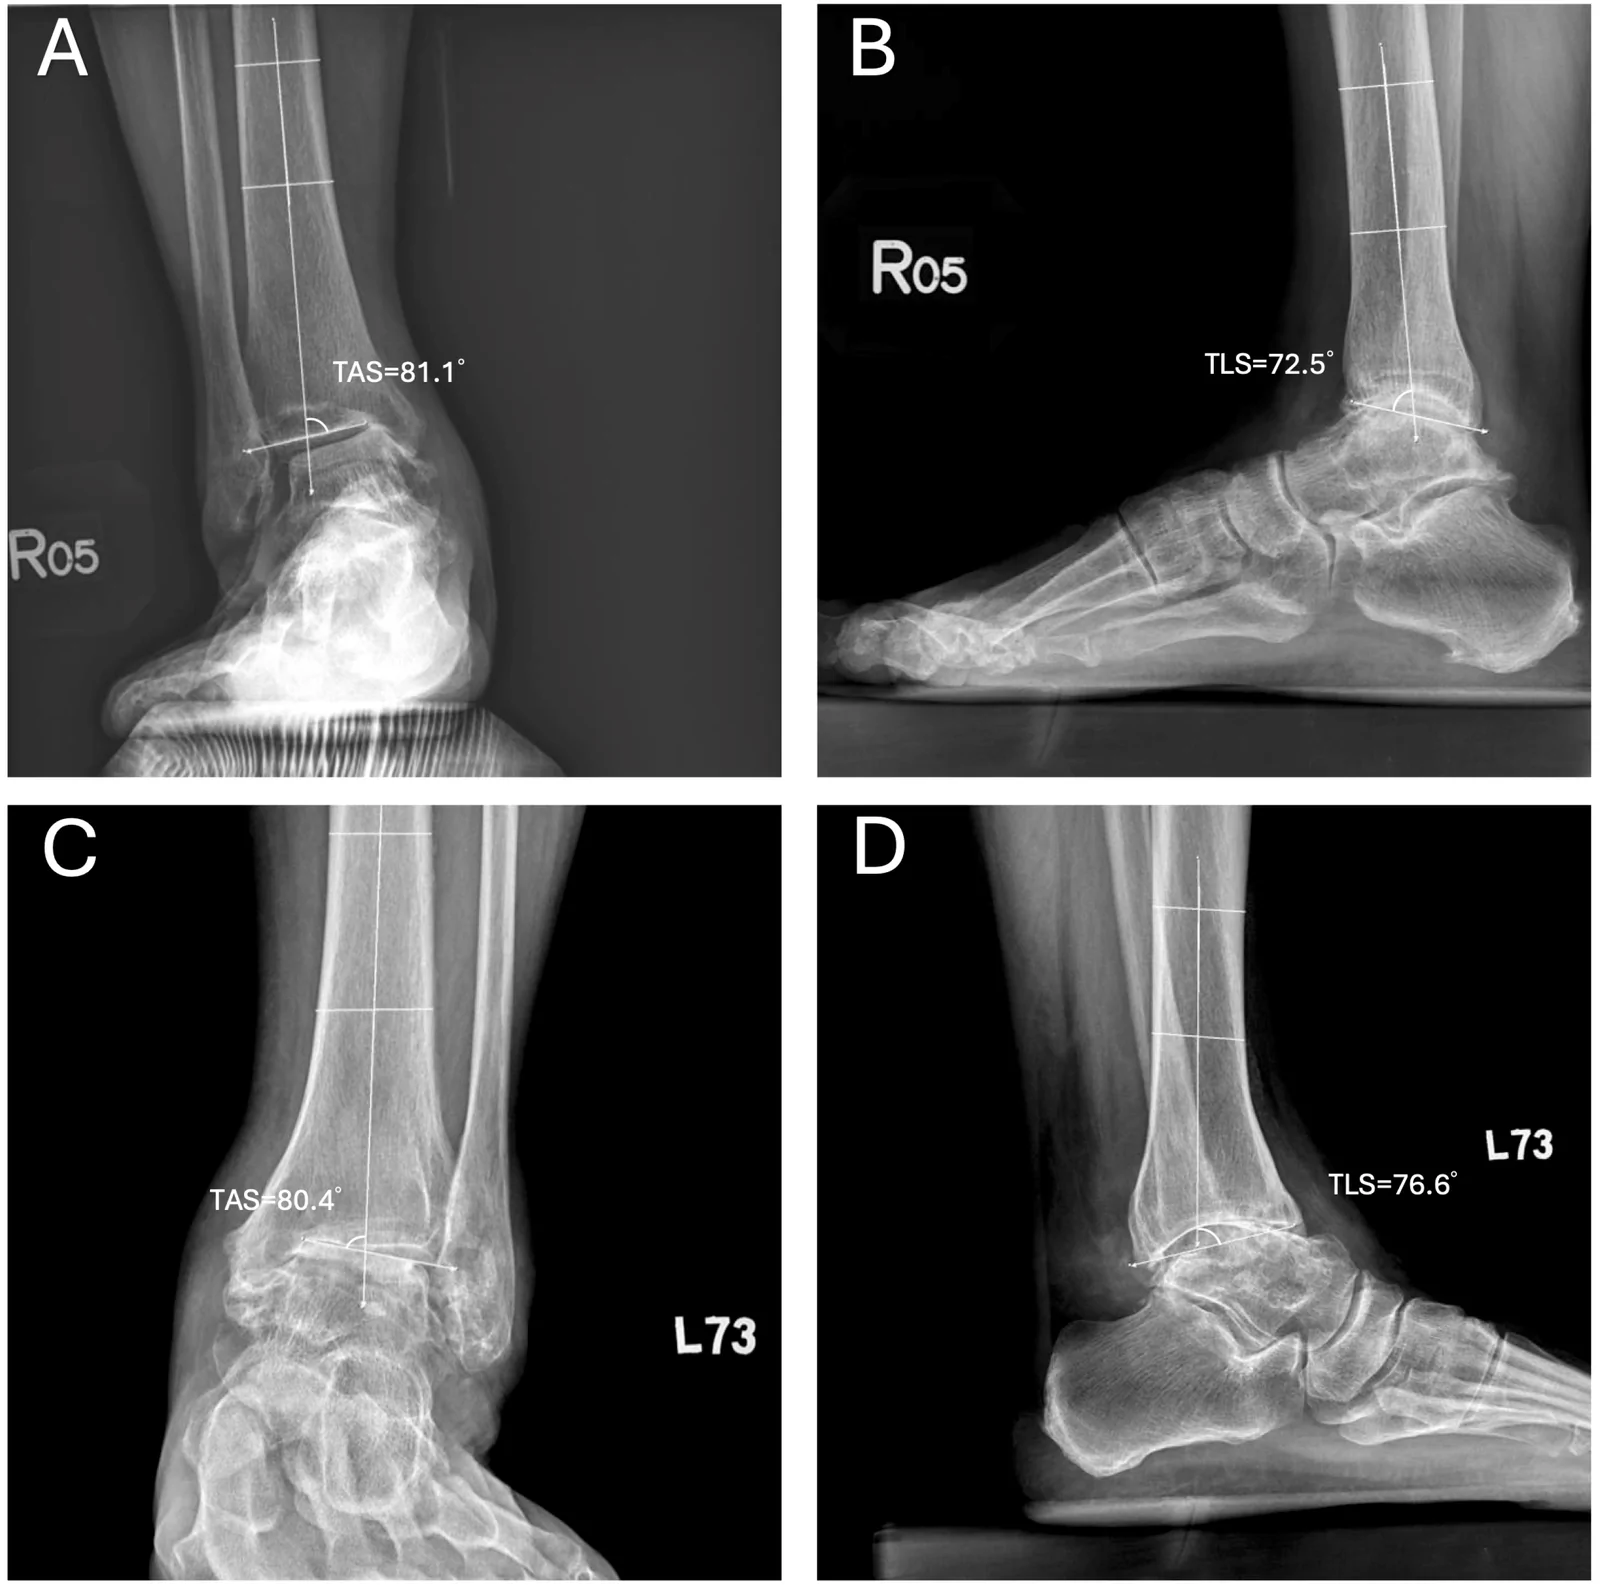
Preoperative weight-bearing radiographs of varus ankle osteoarthritis (Takakura stage IIIa and IIIb). (A) (Stage IIIa) Preoperative AP view demonstrates varus ankle OA with subchondral sclerosis and joint space narrowing, predominantly in the medial gutter. TAS 81.1°, MMA 40.1°, TTA 2.9°, TTS 77.2°. (B) (Stage IIIa) Preoperative lateral view reveals osteophyte formation. TLS 72.5°. (C) (Stage IIIb) Preoperative AP view demonstrates varus ankle OA with subchondral sclerosis and joint space narrowing, predominantly in the medial gutter, dome. TAS 80.4°, MMA 44.9°, TTA 0.3°, TTS 79.5°. (D) (Stage IIIb) Preoperative lateral view reveals talar tilt, subluxation, osteophyte formation. TLS 76.6°.
